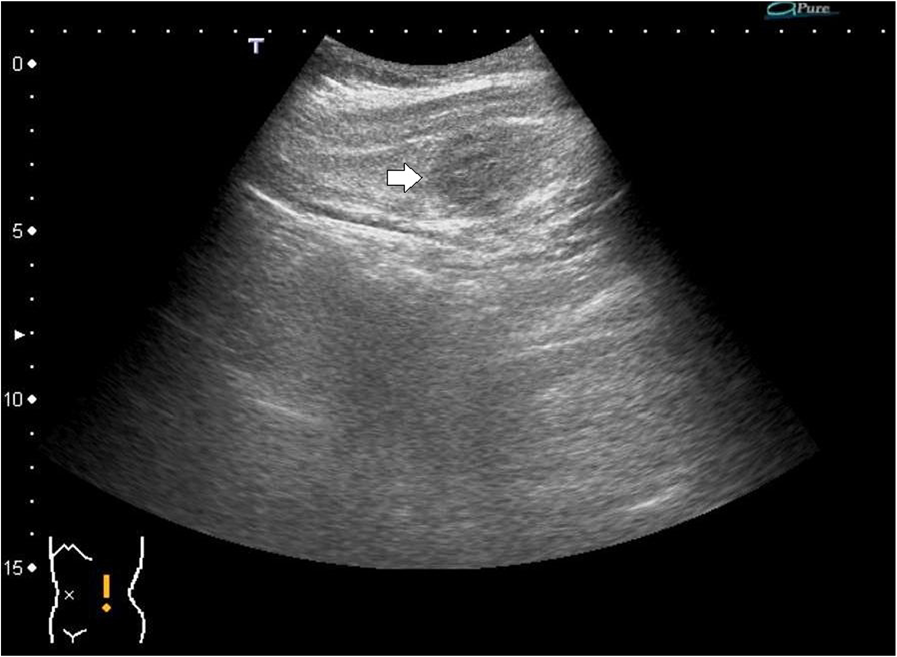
Abdominal ultrasonography (US). An abdominal US showed a heteroechoic mass (white arrow) in the patient’s left lateral abdominal wall.
